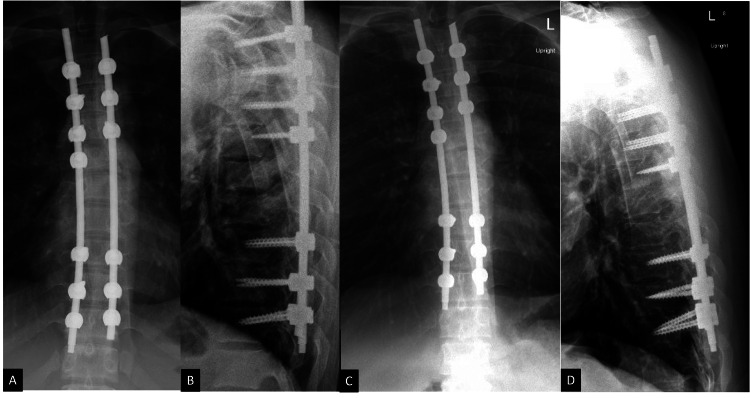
Post-operative plain films at the two-month and 15-month follow-up visits demonstrating stable alignment with no significant interval change. (A) Two-month post-operative upright anteroposterior plain film. (B) Two-month post-operative upright sagittal plain film. (C) Fifteen-month post-operative upright anteroposterior plain film. (D) Fifteen-month post-operative upright sagittal plain film.
